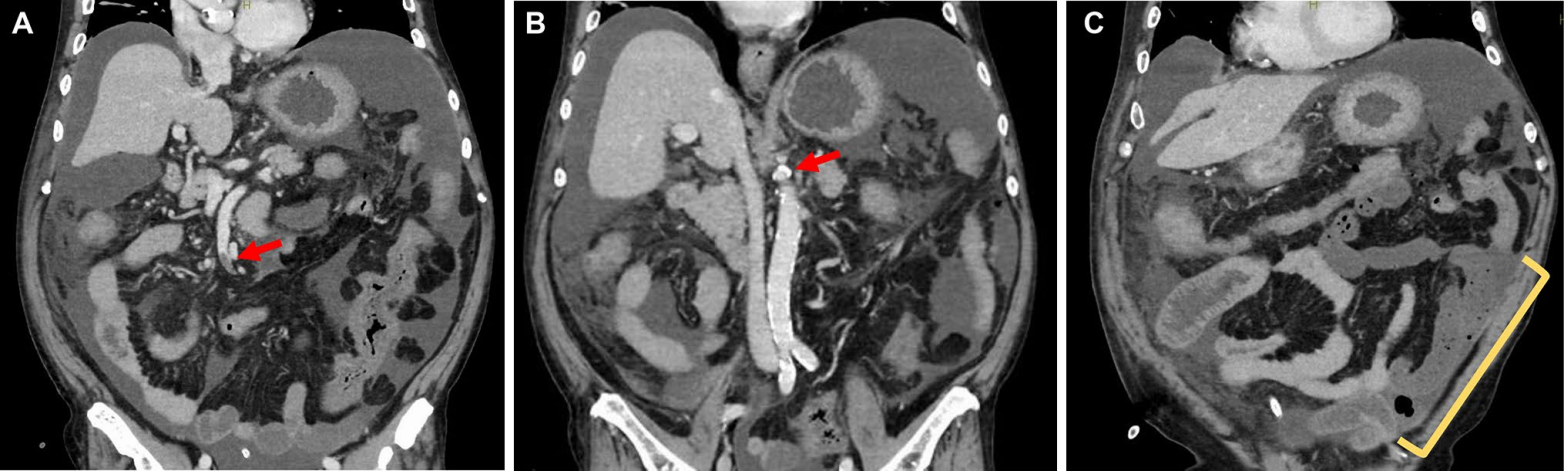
Enhanced computed tomography (CT) findings. Enhanced CT revealed thrombosis of the superior mesenteric artery (SMA) (A), calcification of origin of SMA (B) and intestinal necrosis (C)
